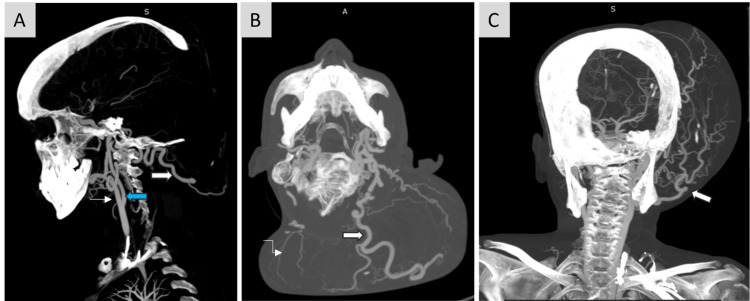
(A) Sagittal, (B) Axial, (C) Coronal maximum intensity projection (MIP) images of CT angiography show dilated tortuous vessels arising predominantly from the terminal maxillary and superficial temporal branches of left ECA (white solid arrow) and a few from the right ECA (curved white arrow) which are seen traversing into and supplying the overlying plexiform neurofibroma. ICA - Blue solid arrow, ECA - White arrow. ICA: internal carotid artery, ECA: external carotid artery
